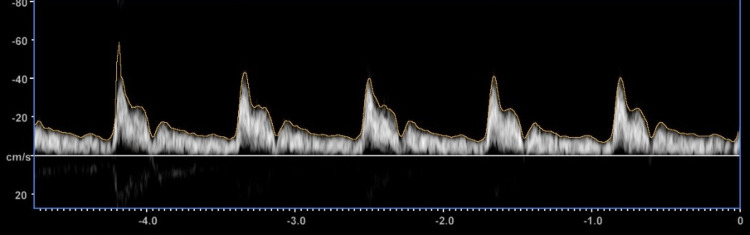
Cervical Doppler showing no relevant changes
